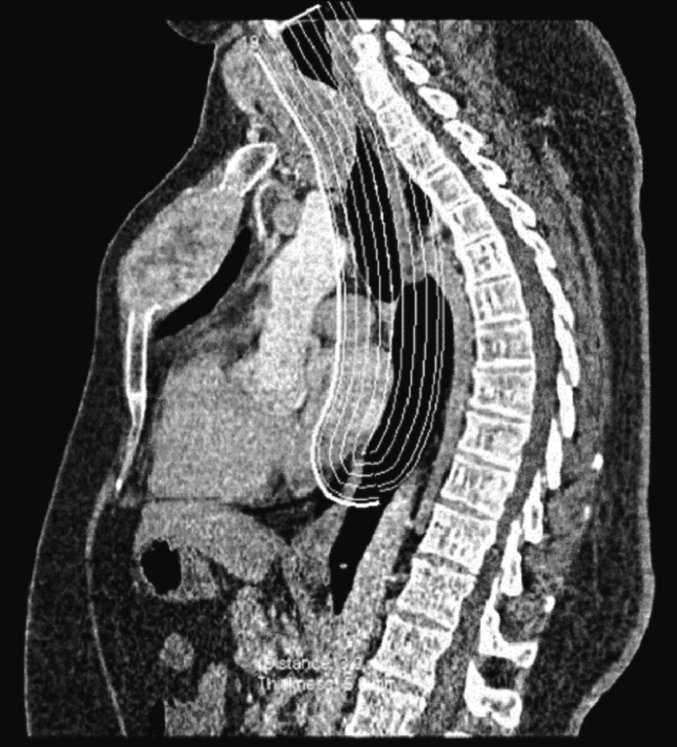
CT scan: showing enlarged nodular thyroid with 2.8 cm retrosternal extension.
